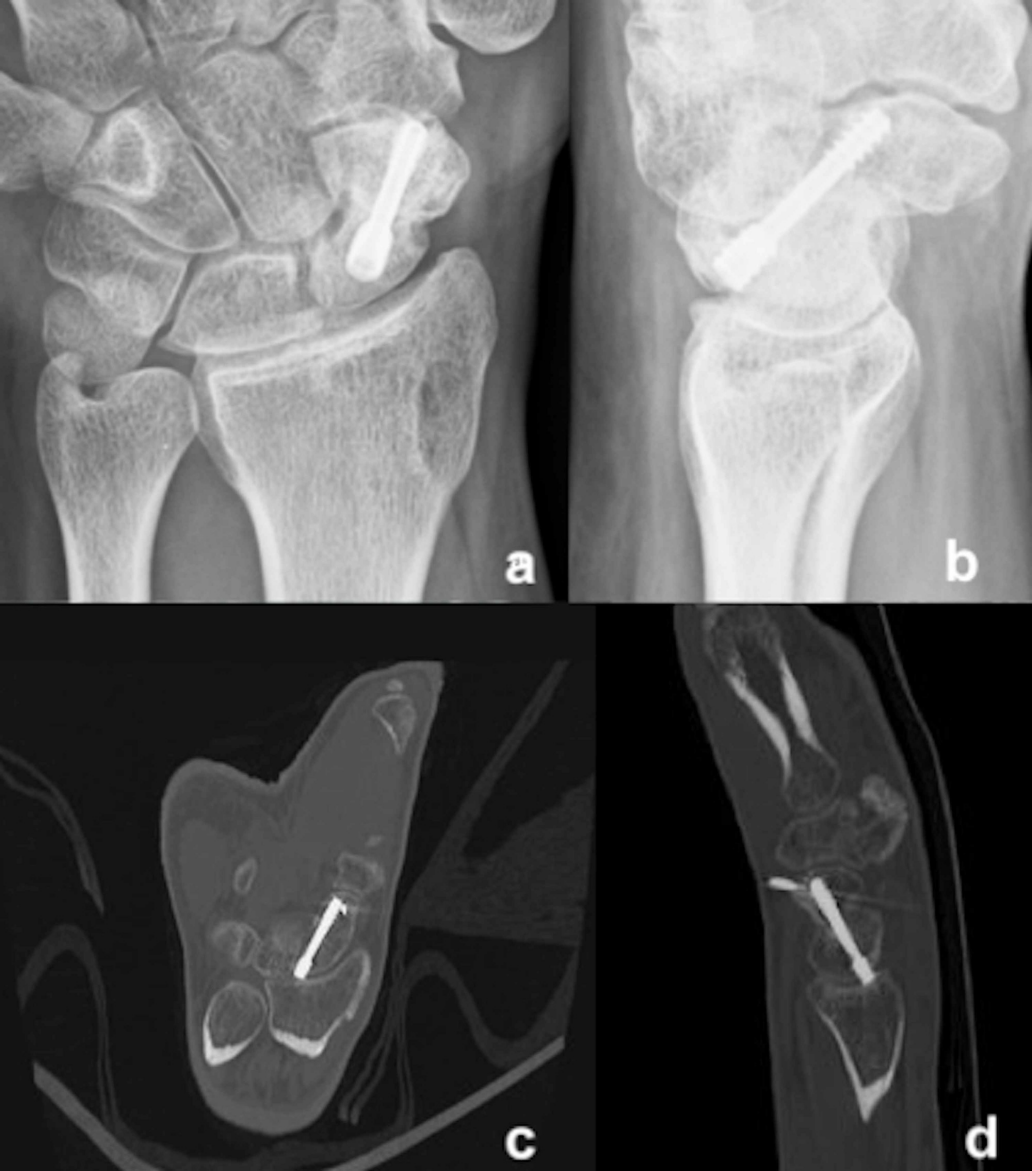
Posteroanterior (a) and lateral (b) radiographs showing good union at weeks after the surgery, CT scan (c, d) shows good union with vascularized bone graft incorporation at the nonunion site
